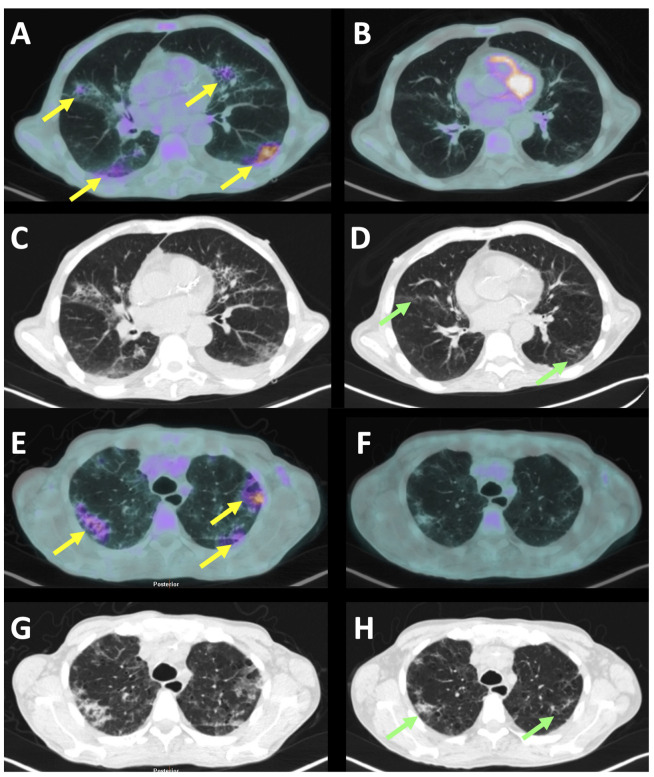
Axial section of the [^18^F]FDG-PET/CT scan of patient A (A–D) and patient B (E–H) before the Tocilizumab infusion (left images) and 14 days after therapy (right images). Yellow arrows indicate the areas of interstitial pneumonia that were metabolically active at the FDG-PET. After therapy, despite the FDG-PET being completely negative, there were still some areas of ground-glass at the CT scan (green arrows). Indeed, the normalization of the CT scan always occurred later than the normalization of the FDG-PET, thus highlighting the importance of monitoring patients’ therapy by FDG-PET.
